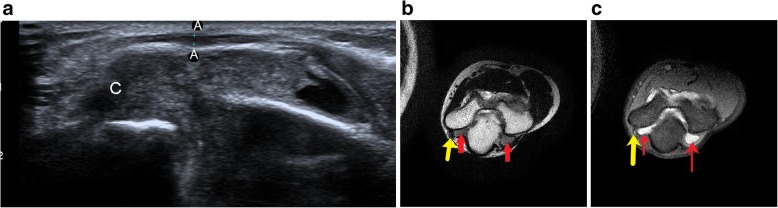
a The ulnar nerve (A) is of normal calibre but is displaced out of the cubital tunnel due to underlying hypoechoic collection (C) within the joint cavity. b T1-weighted axial image at the level of cubital tunnel shows hypoechoic ulnar nerve (yellow arrow) with surrounding isointense significantly thickened synovium (red arrows). c PD FS image shows isointense ulnar nerve (yellow arrow) with normal calibre with surrounding hyperintense synovial thickening (red arrows) and minimum joint effusion. On further laboratory investigation, the patient was diagnosed to be a case of rheumatoid arthritis
